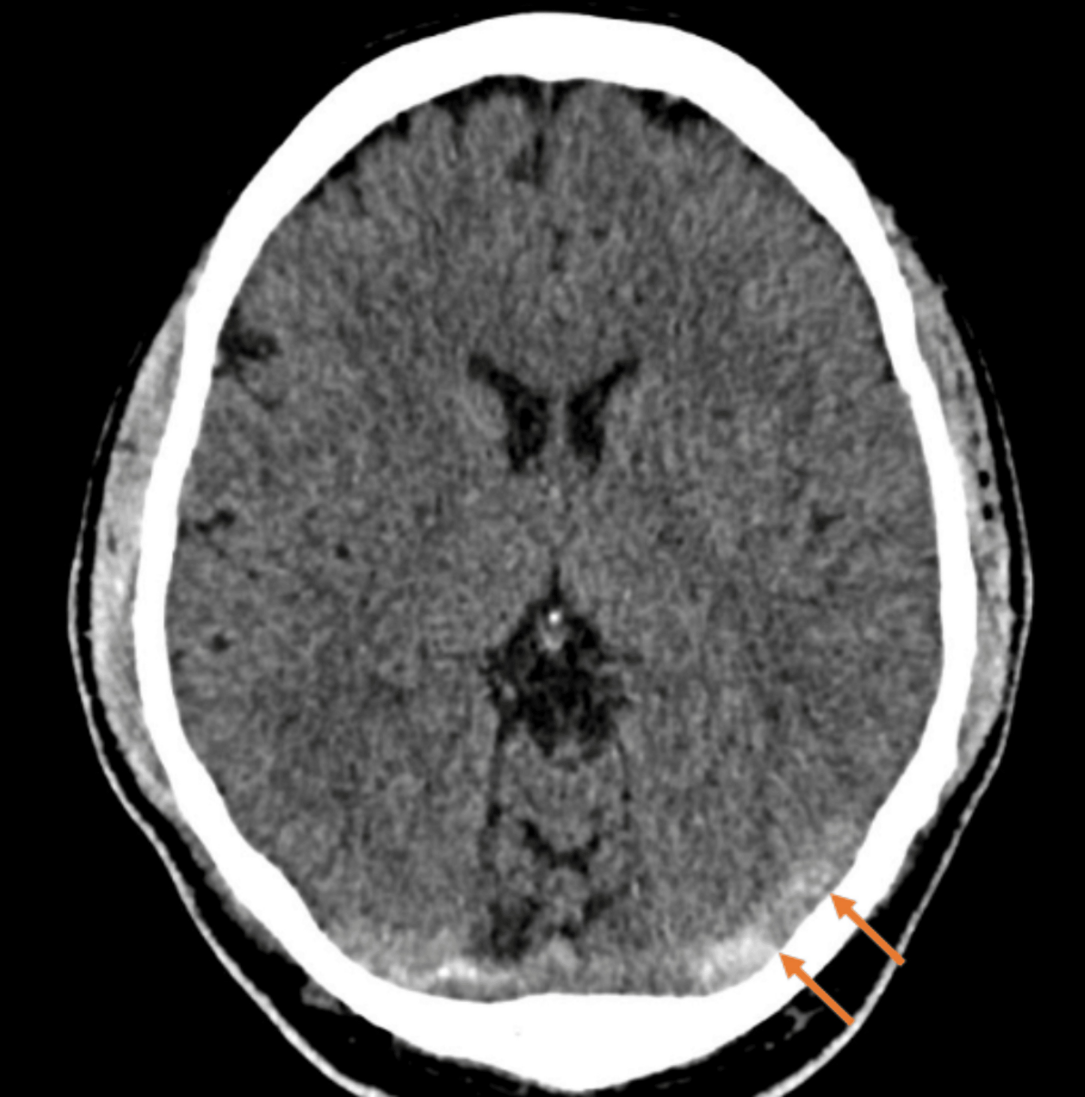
A non-enhanced CT image (axial view) showing areas of abnormal hyperattenuation (orange arrows) that are consistent with CVST in the left transverse sinus CVST: cerebral venous sinus thrombosis; CT: computed tomography
